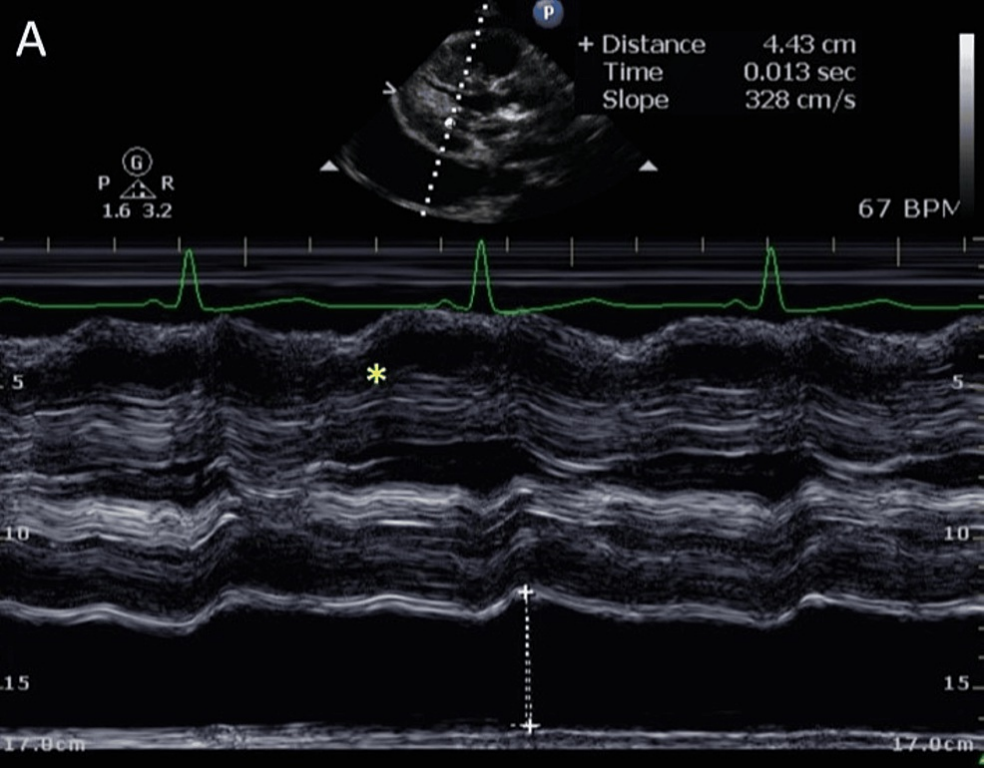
Transthoracic echocardiogram M-mode depicting right ventricle collapse in diastole (yellow star).
